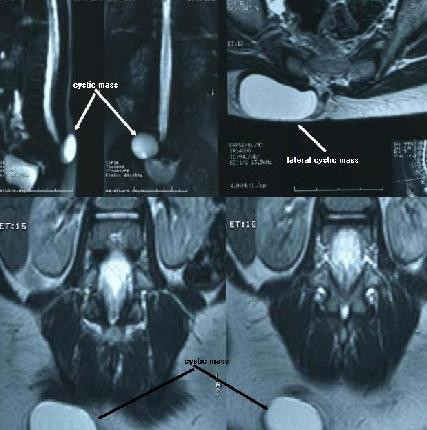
Magnetic resonance image of the patient's lumbosacral spine. The sagittal, axial and coronal view shows lateral sacral meningocele.
